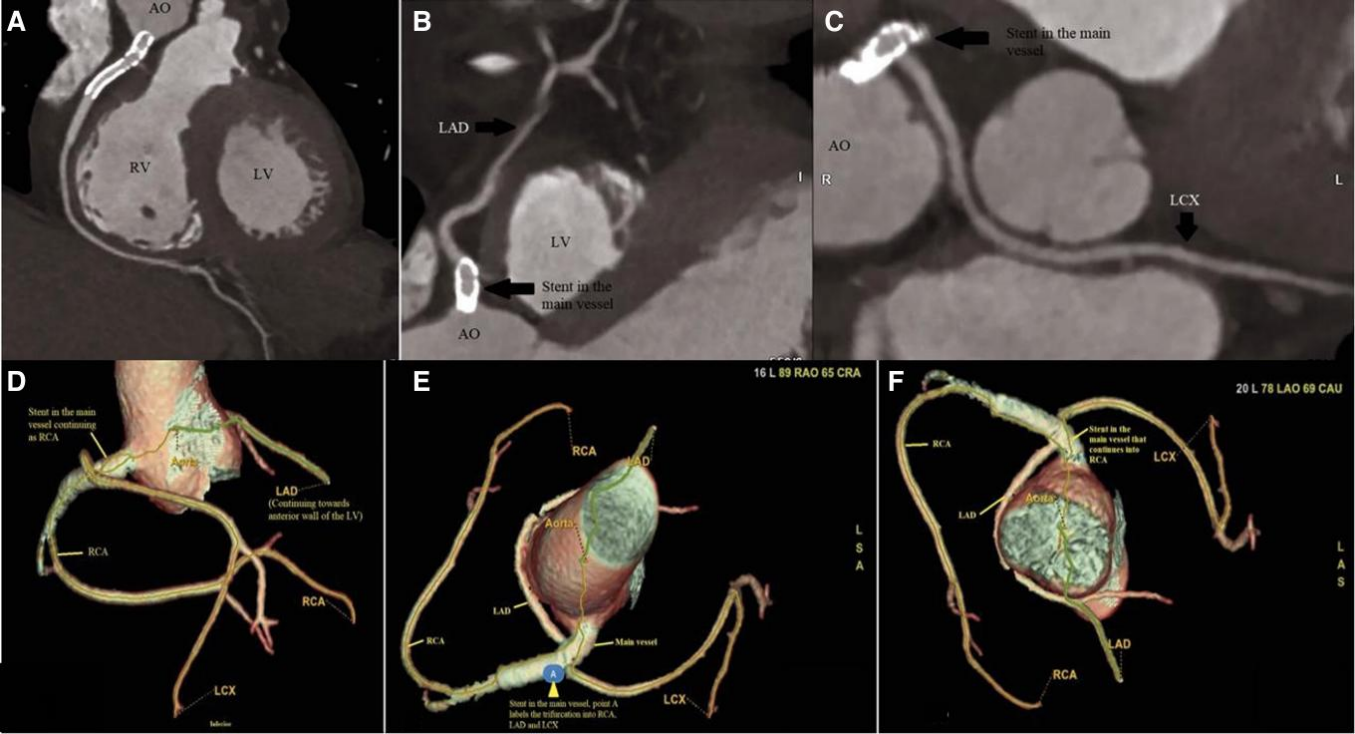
CT reconstruction, including three-dimensional views, of coronary arteries arising from the right coronary artery. (*A*) RCA showing patent stent in the ostio-proximal portion of the artery followed by normal course of a dominant RCA. (*B*) LCx arises from the right coronary artery and supplies posterolateral wall and has a benign course. (*C*) The LAD arising from the RCA at the ostial portion of which the deployed stent is visible and the vessel supplies anterior wall and anterior septum of the LV. (*D*) Right anterior oblique view demonstrating the stented main vessel, LAD arising from the right coronary artery and coursing anteriorly towards the anterior wall of the left ventricle. (*E*) Superior view of the aorta showing the single coronary ostium at the right coronary cusp, with the stented main vessel visible; point A marks the site of trifurcation into the RCA, LAD, and LCx. (*F*) Additional superior view of the aorta further delineating the course of the coronary arteries relative to the great vessels.
